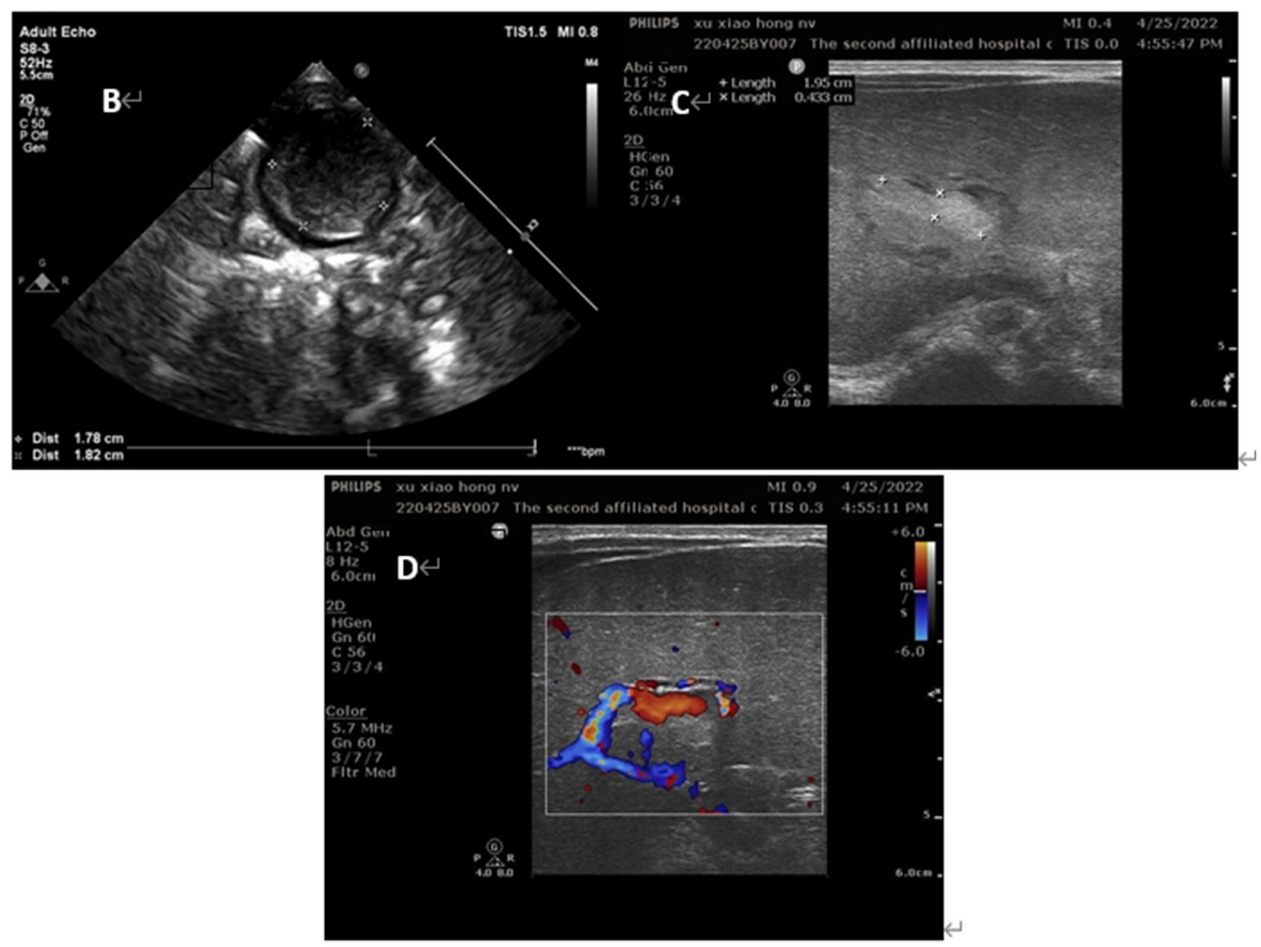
Ultrasound images of the patients on day 2 of life (B) umbilical vein thrombus at day 2; (C) portal vein thrombus at day 2; (D) the blood flow signal decreased in the lumen of portal vein thrombosis at day 2.
